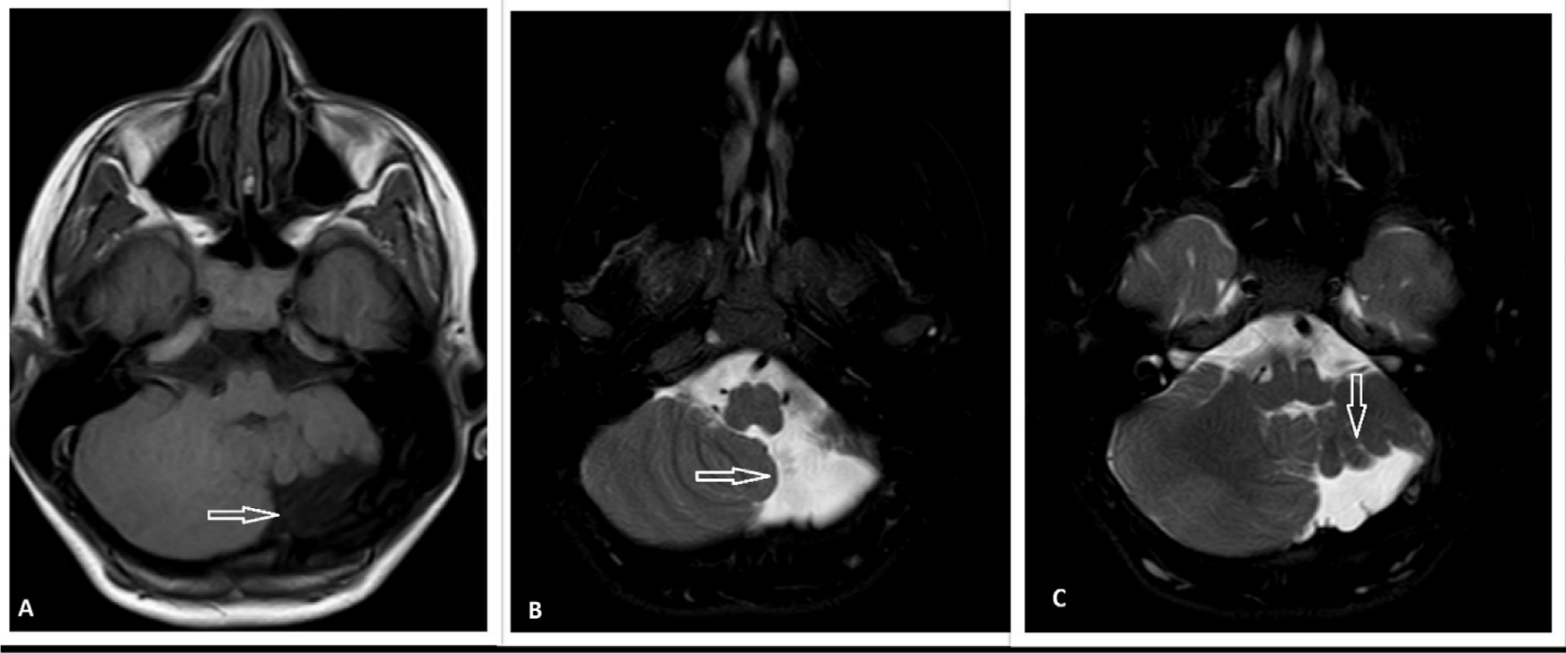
Axial T1W (A), T2W (B, C) sequences brain MRI of left cerebellar hypoplasia. There is marked hypoplasia of the left cerebellar hemisphere (arrows) and normal volume of the right cerebellum. No signal abnormality or gliosis in the left cerebellum is seen. The right cerebellar hemisphere is normal in bulk and signal intensity. The normal bulk of the vermis and brain stem can also be seen.
